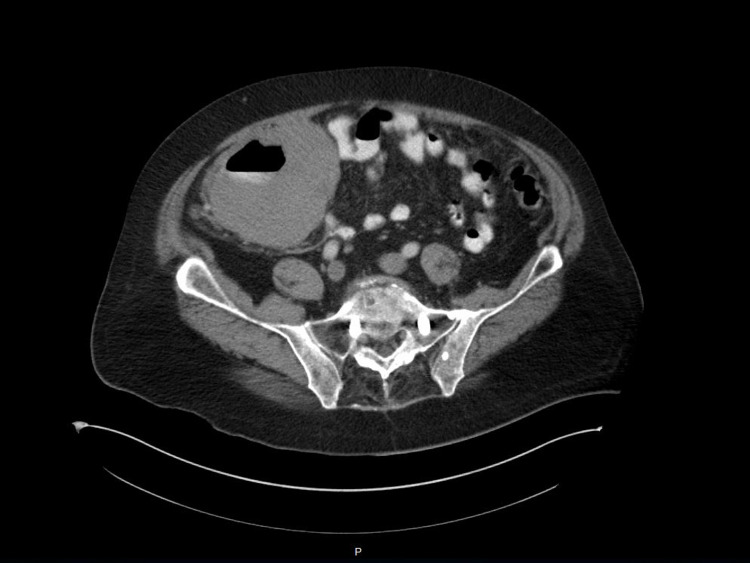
CT scan of the abdomen showing large nearly circumferential mass at the cecal and terminal ileal. CT: computed tomography
